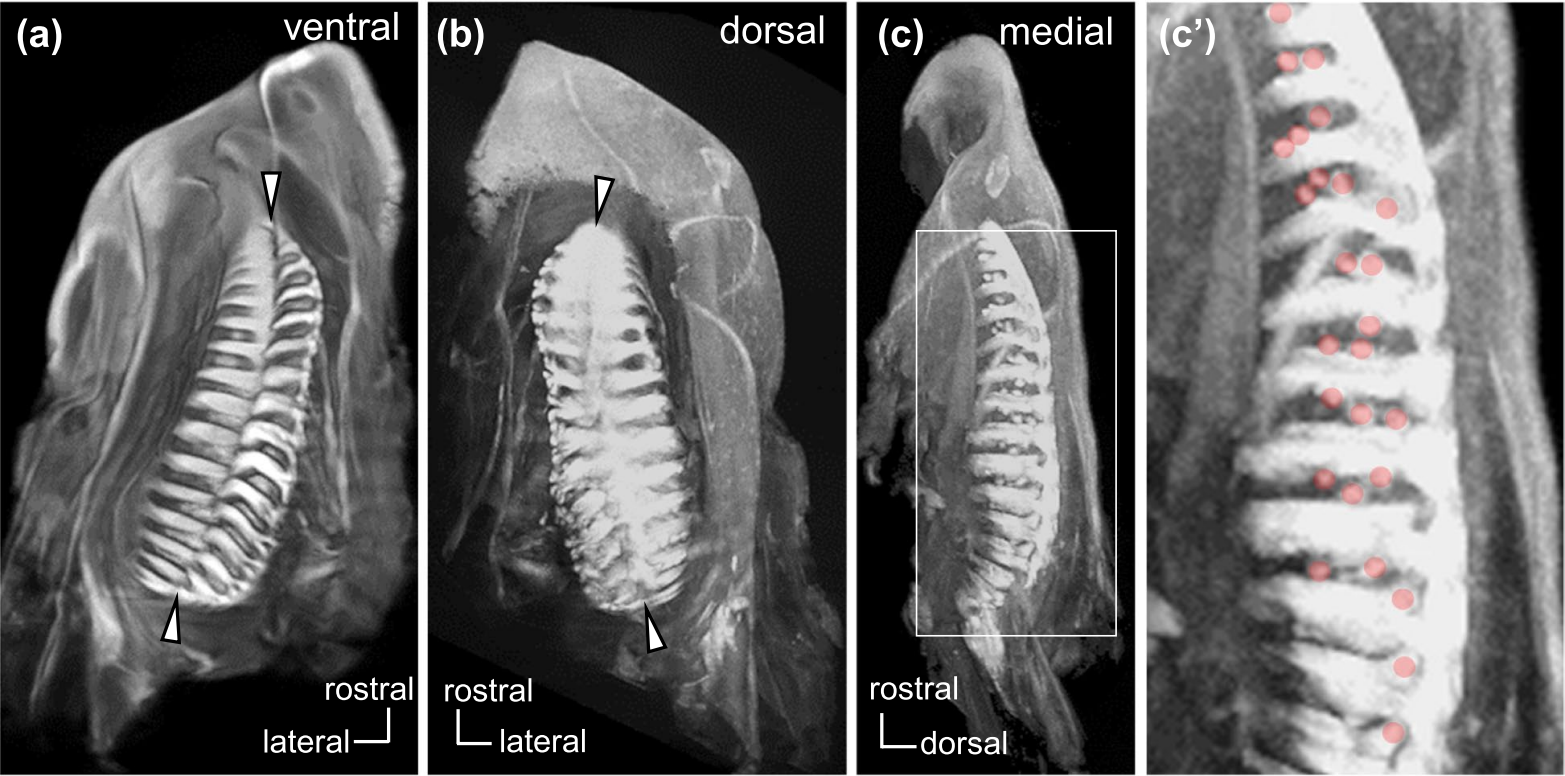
Diffusible iodine-based contrast-enhanced computed tomography images of the olfactory organ in *P. aethiopicus*. The right olfactory organ viewed from the ventral (a), dorsal (b) and medial (c) aspects. Higher magnification view of (c) is shown in (c’). Lamellae were arranged on the medial and lateral sides of the midline raphe (arrowheads). Recesses were abundantly present at the base of lamellae. The recesses are highlighted by red-shaded circles in (c’)
